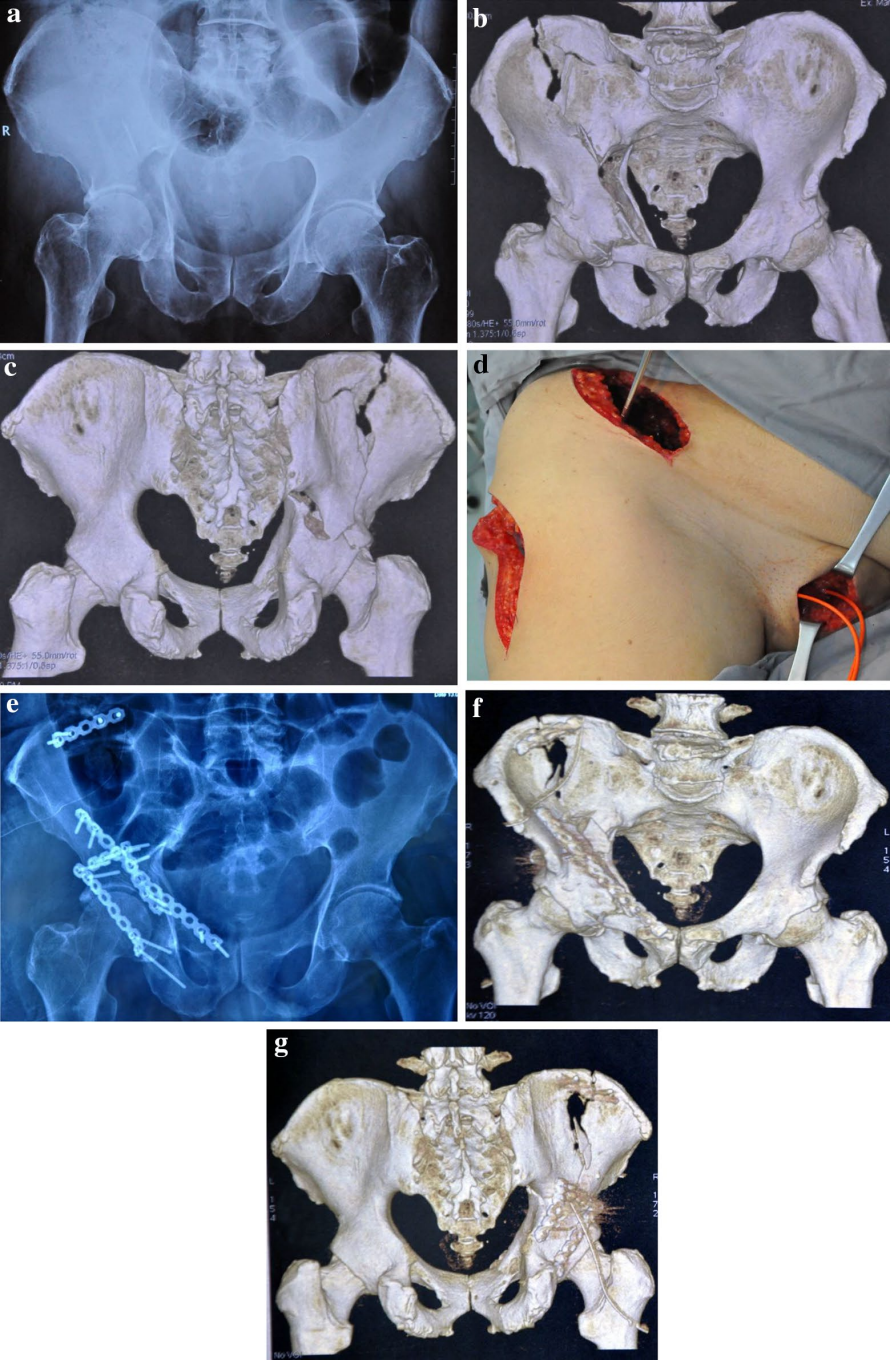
a Preoperative diagnostics of an acetabular fracture in a 65-year-old man by falling injury. b, c 3D CT scan demonstrating both-column acetabular fractures. d Intraoperative view of the modified ilioinguinal approach combined with a K–L approach. e Postoperative anteroposterior radiograph showing anatomic reduction of the acetabular fractures. f, g Postoperative 3D CT scan showing anatomic reduction of the acetabular fractures
